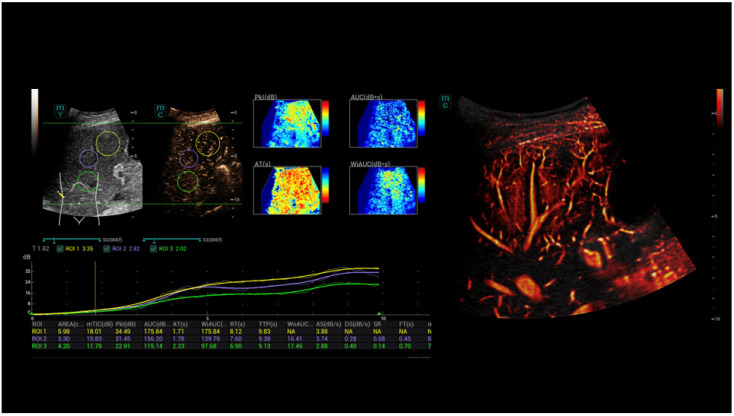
SR CEUS and CEUS perfusion and after injection of 1.4 mL ultrasound contrast agent with irregular hyperenhancement of the liver and early nodular enhancement of high-flow haemangiomas and micro-shunts. Visualisation of the early time–intensity curve (TIC) hyperenhancement of the shunts by dynamic SRCEUS (red and orange), as well as by colour maps of peak enhancement (PKI) and wash-in area-under-the-curve (WiAUC) (red and yellow).
